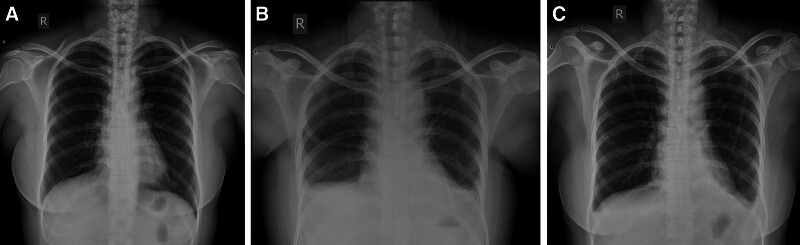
Chest X-rays of case 2: (A) preoperative chest X-ray was normal. (B) On postoperative day 1 (POD1), bilateral pleural effusion was observed: blunting of bilateral costophrenic angles. (C) Predischarge chest X-ray on POD9 after administration of octreotide showed lung re-expansion, sharp right costophrenic angle, and slightly blunted left costophrenic angle.
